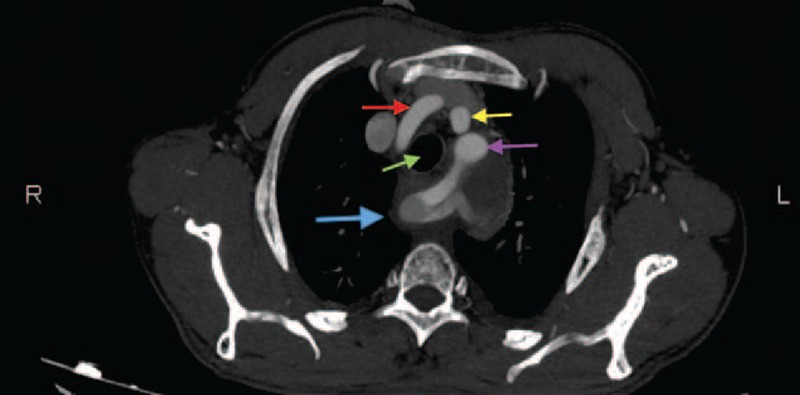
CT scan image. Axial view. LCCARS and Stanford B aortic dissection. (Blue arrow, dissected aberrant right subclavian artery; red arrow, right common carotid artery; purple arrow, left subclavian artery; yellow arrow, left common carotid artery; green arrow, trachea.) CT = computed tomography, LCCARS = left aortic arch with common origin of the carotid arteries, left subclavian artery, and aberrant right subclavian artery.
